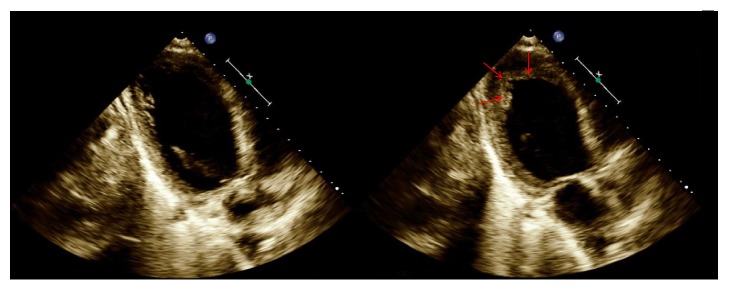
TTE apical two-chamber view of left ventricle in End Diastole (*left*) and End Systole (*right*), demonstrating poor ventricular compression and low EF. r-TTC pattern is indicated by contraction of ventricular apex (arrows) and hypokinesis of base during systole.
